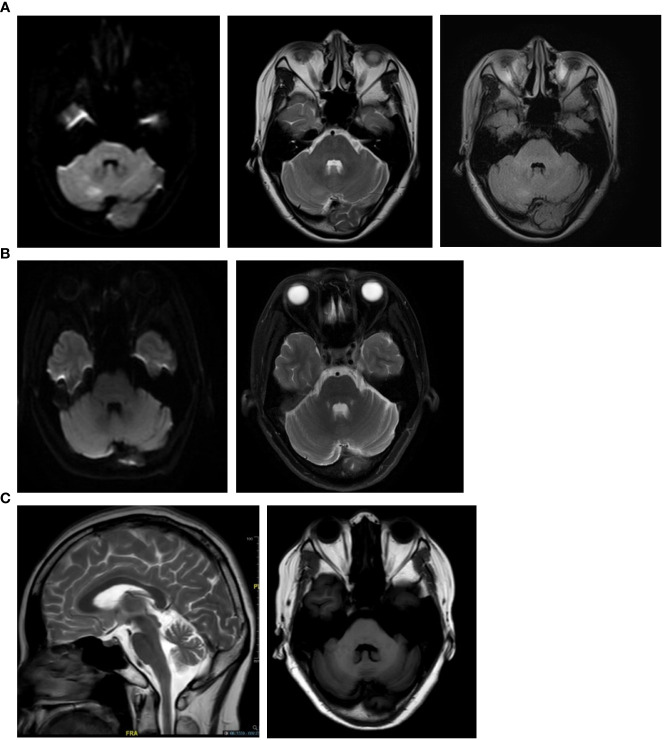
Brain magnetic resonance imaging of the anti-EEF1D-positive patient in case 1. (A) Brain MRI scans conducted two months after disease onset (May 2019) showed multiple hyperintense lesions in the cerebellum of the patient in case 1. (B) One month later (June 2019), follow-up brain MRI scans showed that the lesions in the cerebellum of the patient in case 1 had disappeared. (C) MRI conducted in October 2019 showed cerebellar atrophy.
